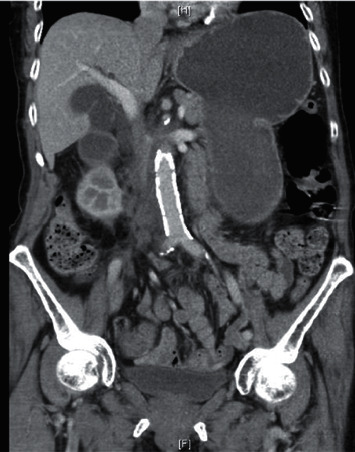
Coronal view of CT scan of the abdomen and pelvis showing wall thickening of the 2nd and 3rd parts of the duodenum and proximal distention of the 1st part of the duodenum and stomach.
